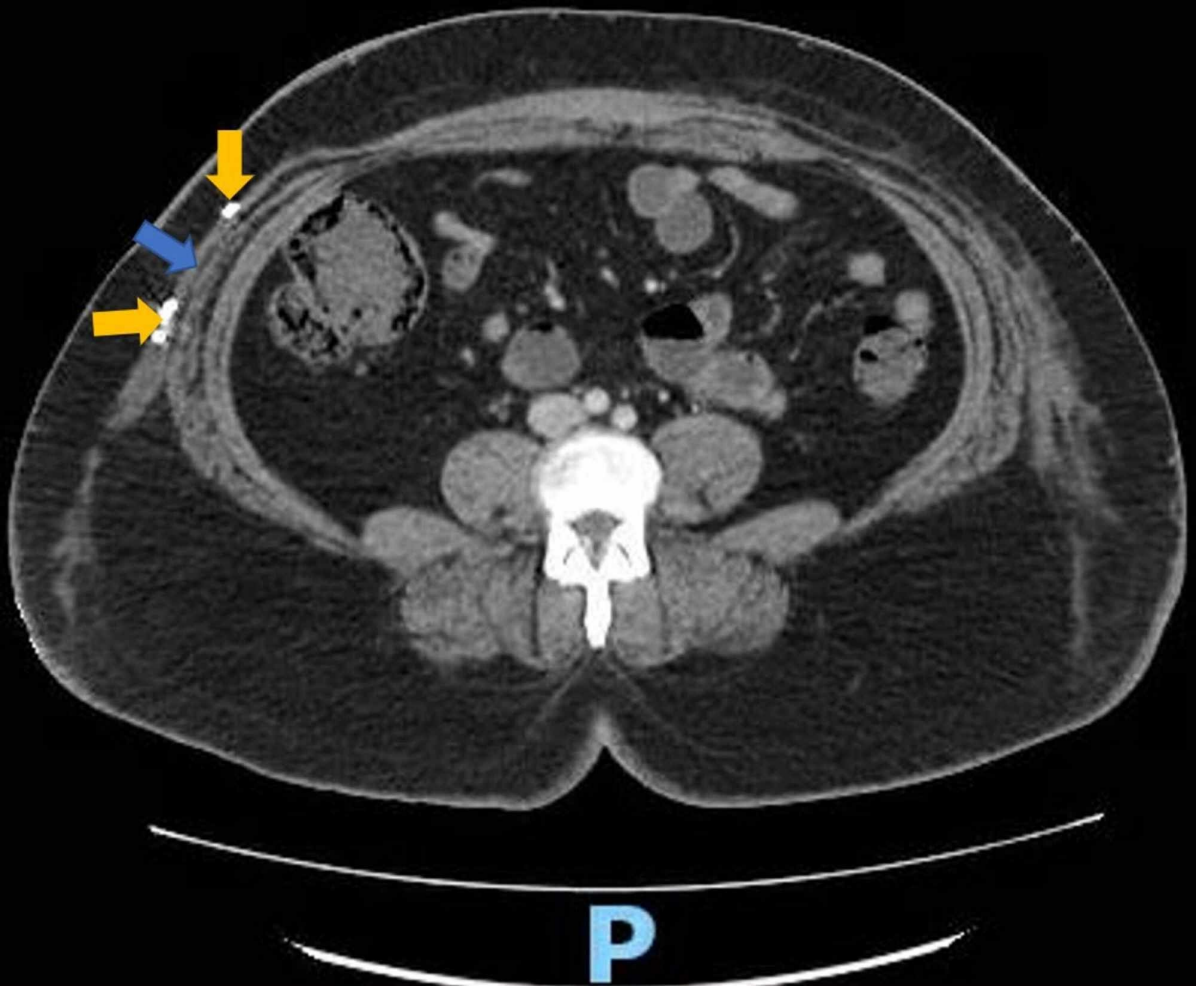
CT scan: transverse view of abdominal cavity. This CT scan was performed after placement of the pigtail catheter. The right-sided fluid collection has been drained (blue arrow) and the ends of the pigtail catheter (golden arrows) can be seen traversing the cavity. The letter P denotes posterior orientation of the patient.
